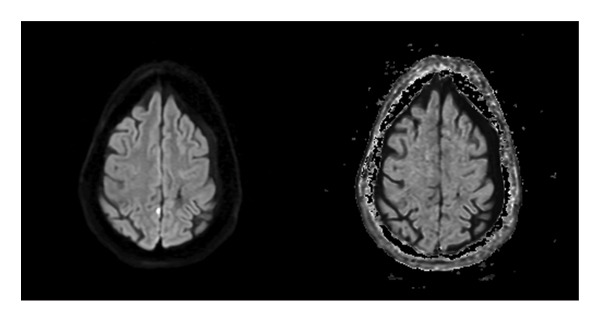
MRI brain, axial diffusion‐weighted (b1000) with corresponding ADC map.
